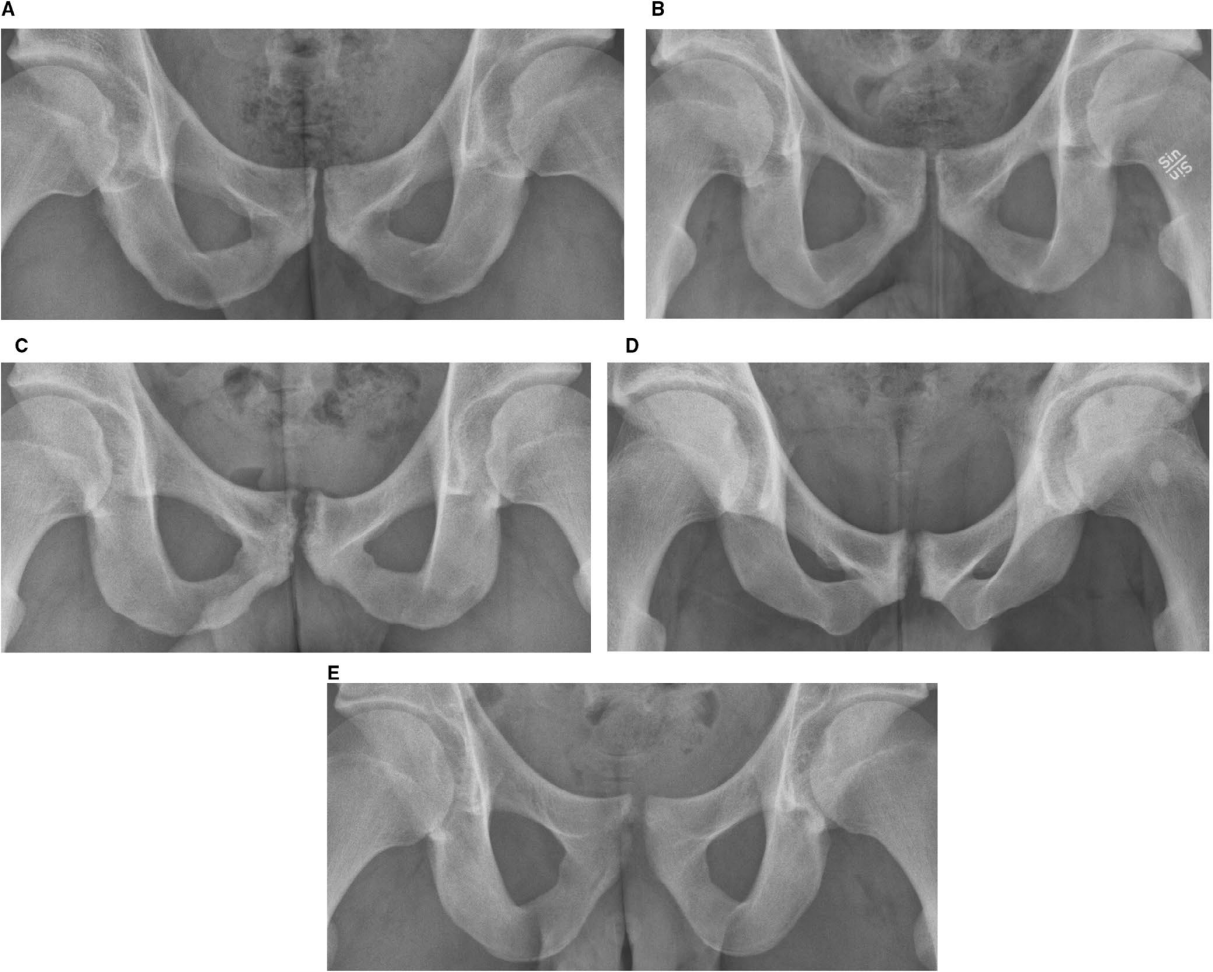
Examples of anteroposterior radiographs of athletes with and without groin pain. (A) Symptomatic football player: 26 years, 178 cm, 72 kg, dominant leg: Right, bilateral groin pain, pain duration: 2 months, worst pain last week 8, 5SST: 8, HAGOS Pain 100, Symptoms 66.7, ADL 81.3, Sport 37.5, PA 25, QoL 75; Clinical Entities: Bilateral adductor‐related, bilateral iliopsoas‐related, bilateral inguinal‐related, and pubic‐related groin pain. Pubic‐related radiographic findings total = 0 and JSW: 3 mm. (B) Symptomatic football player: 25 years, 182 cm, 75 kg, dominant leg: Right, right‐sided groin pain, pain duration: 6 weeks, worst pain last week: 7, 5SST: 8, HAGOS Pain 78.1, Symptoms 54.2, ADL 68.8, Sport 42.7, PA 0.0, QoL 43.8; Clinical Entities: Right‐sided adductor‐related groin pain, right‐sided inguinal‐related groin pain. Pubic‐related radiographic findings total = 0 and JSW: 4 mm. (C) Asymptomatic football player: 23 years, 180 cm, 79 kg, dominant leg: Right, HAGOS Pain 100, Symptoms 100, ADL 100, Sport 100, PA 100, QoL 100, Pubic‐related radiographic findings total = 14: Bilateral bone lucency, ELC, central/superior ELC; right‐sided cysts, bilateral proliferation, superior proliferation, central proliferation, inferior proliferation; bilateral sclerosis; JSW = 2 mm. (D) Symptomatic football player: 24 years, 188 cm, 80 kg, dominant leg: Right, bilateral groin pain, pain duration: 48 months, worst pain last week: 9, 5SST: 6, HAGOS Pain 37.5, Symptoms 29.2, ADL 31.3, Sport 29.2, PA 12.5, QoL 18.8; Clinical Entities: Bilateral adductor‐related, bilateral iliopsoas‐related, left inguinal‐related, and pubic‐related groin pain; Pubic‐Related Radiographic Finding total = 13: Bilateral findings of Bone lucency, ELC, central/superior ELC, proliferation, superior proliferation, central proliferation, and sclerosis. Left sided inferior proliferation, fragmentation, inferior fragmentation. JSW 1 mm. (E) Asymptomatic non‐football athlete: 19 years, 184 cm, 72 kg, dominant leg: Left, HAGOS Pain 100, Symptoms 100, ADL 100, Sport 100, PA 100, QoL 100, Pubic‐related radiographic findings: Bilateral bone lucency, ELC, central/superior ELC; left‐sided cysts, bilateral proliferation, superior proliferation; right‐sided central proliferation, and JSW = 2 mm.
